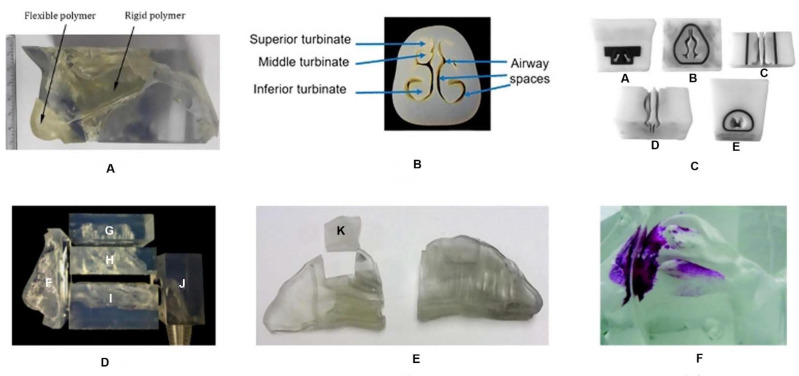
Examples of different nasal casts: (A) 7-year-old child; (B) MRI image of a 12-year-old child; (C) made from CT-scan of an adult; (CA) nostrils, (CB) nasal vestibule, (CC) lower turbinates, (CD) middle and upper turbinates, (CE) nasopharynx; (D) CT-scan of a patient, (DF) anterior region, (DG) upper turbinates, (DH) middle turbinates, (DI) lower turbinates, (DJ) nasopharynx; (E) MRI image of a 53-year-old man, K—olfactory region; (F) silicone transparent commercial cast with Sar-gel (adapted from [90], with permission from Elsevier).
